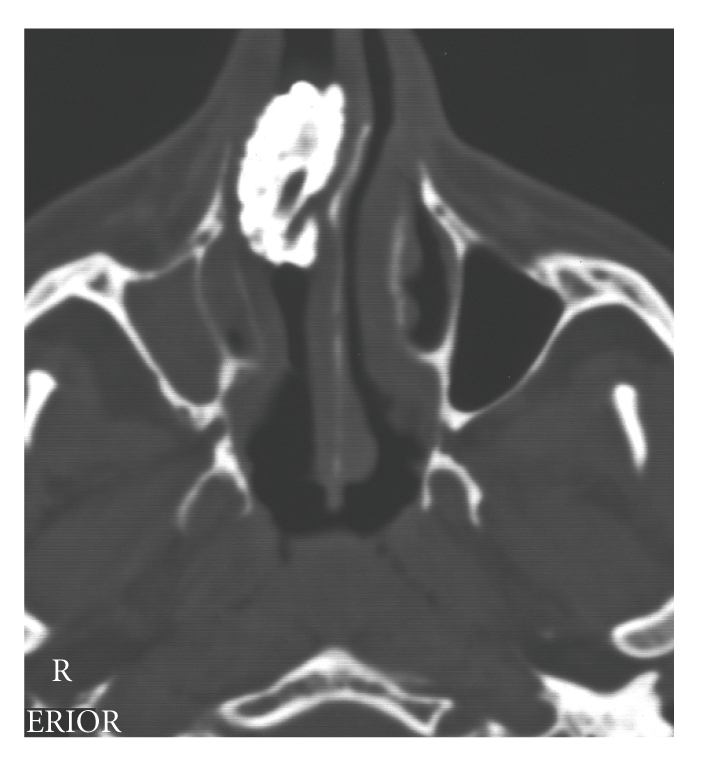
Axial CT scan showing the rhinolith in the right nasal cavity, with consecutive shadowing of the right maxillary sinus.
